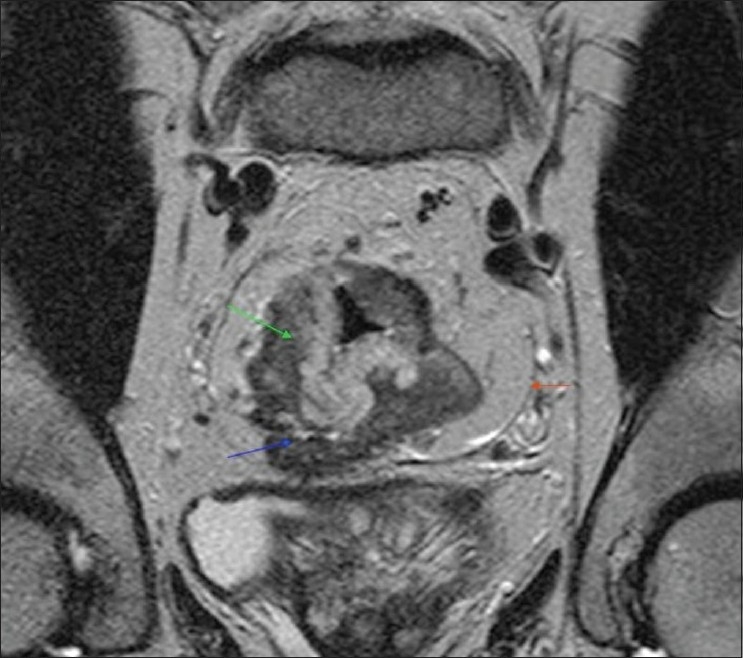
Coronal T2 spin echo MRI showing bulky rectal tumor (green arrow), CRM (red arrow) and its involvement by the tumor (blue arrow)
